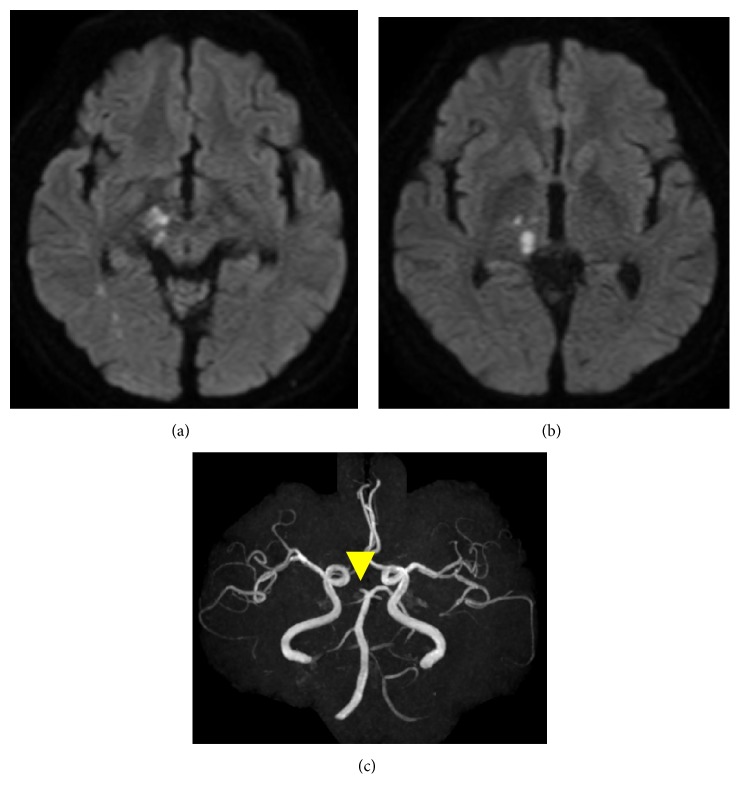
Head MRI performed about 24 hours after onset showed right occipital lobe, thalamus, cerebral crus, and midbrain fresh infarction (a, b). MRA showed right P2 (arrow head) and left SCA (superior cerebellar artery) occlusion (c).
